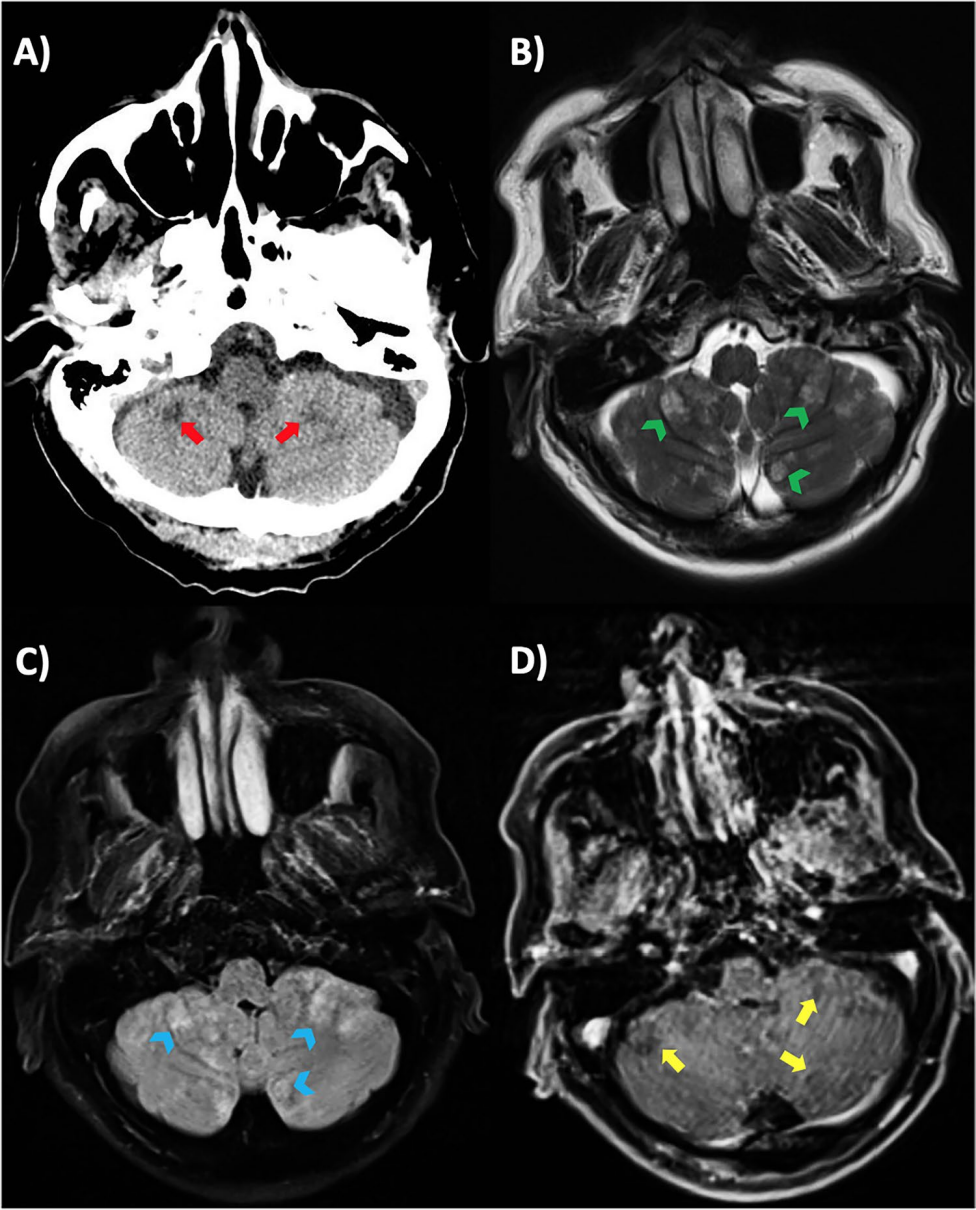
A CT brain showing cerebellar hypodense lesions (arrow); B and C axial T2-weighted MRI sequences showing hyperintense focal parenchymal masses (arrowheads); D post-gadolinium MRI sequence showing no contrast enhancement (arrows)
